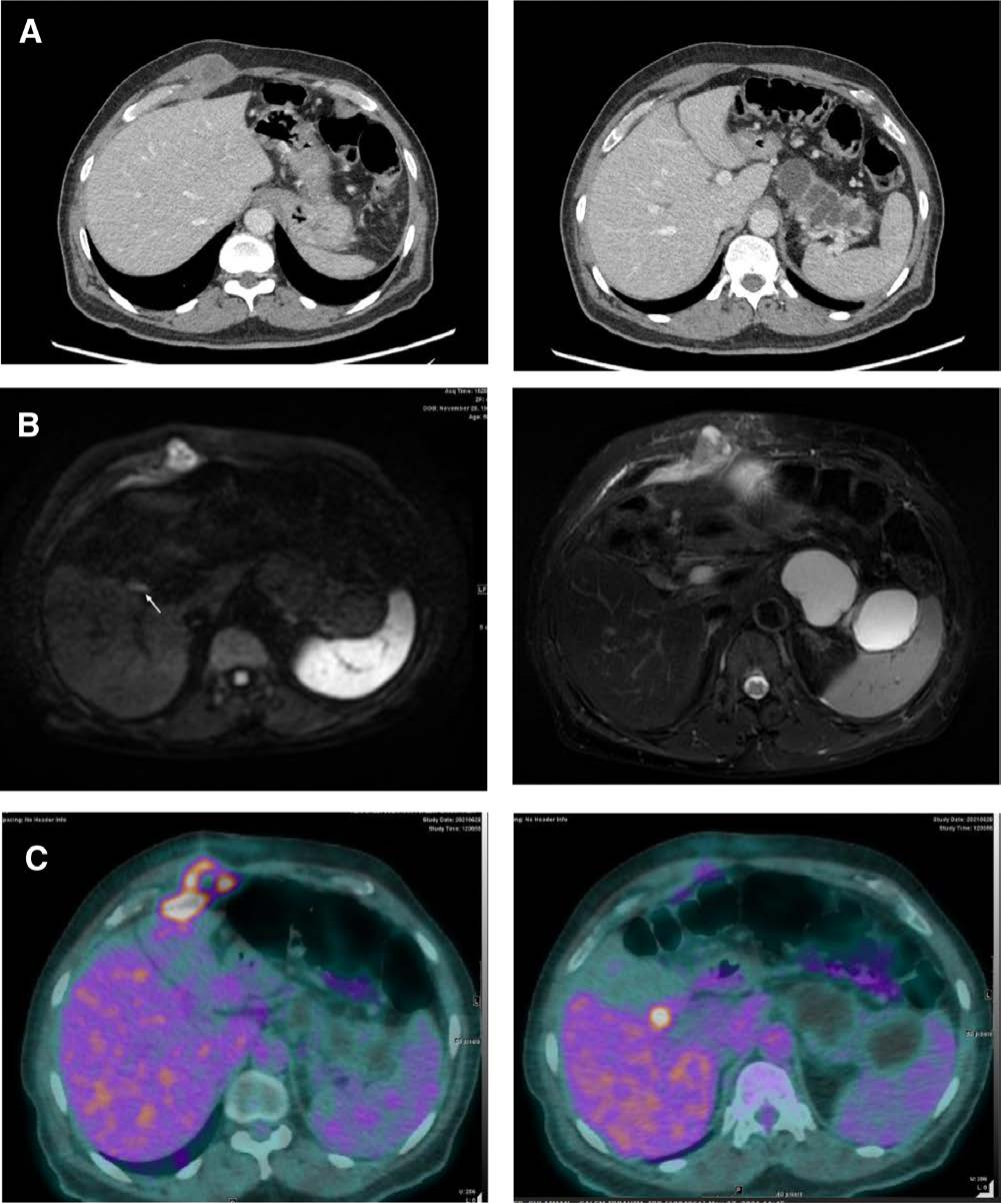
(A) Axial computed tomography CT scan with IV- contrast shows an anterior abdominal wall low density well-defined soft tissue mass at the right costo-chondoral area. The pancreas shows a significantly dilated duct as a sequelae of wall-off necrosis at the pancreatic neck secondary to pancreatitis. (B) Magnetic resonance imaging RI study of the liver in T2W and DW images show a focal soft tissue lesion of high signal intensity in T2W with diffusion restriction. Another tiny small linear high T2W signal and diffusion restriction are seen in the gallbladder bed. (C) Positron emission tomography computed tomography PET CT scan shows heterogeneous fluorodeoxyglucose (FDG) uptake in the right anterior abdominal wall small focus of intense FDG uptake in the gallbladder bed. CT = computed tomography, FDG = fluorodeoxyglucose, PET = positron emission tomography.
